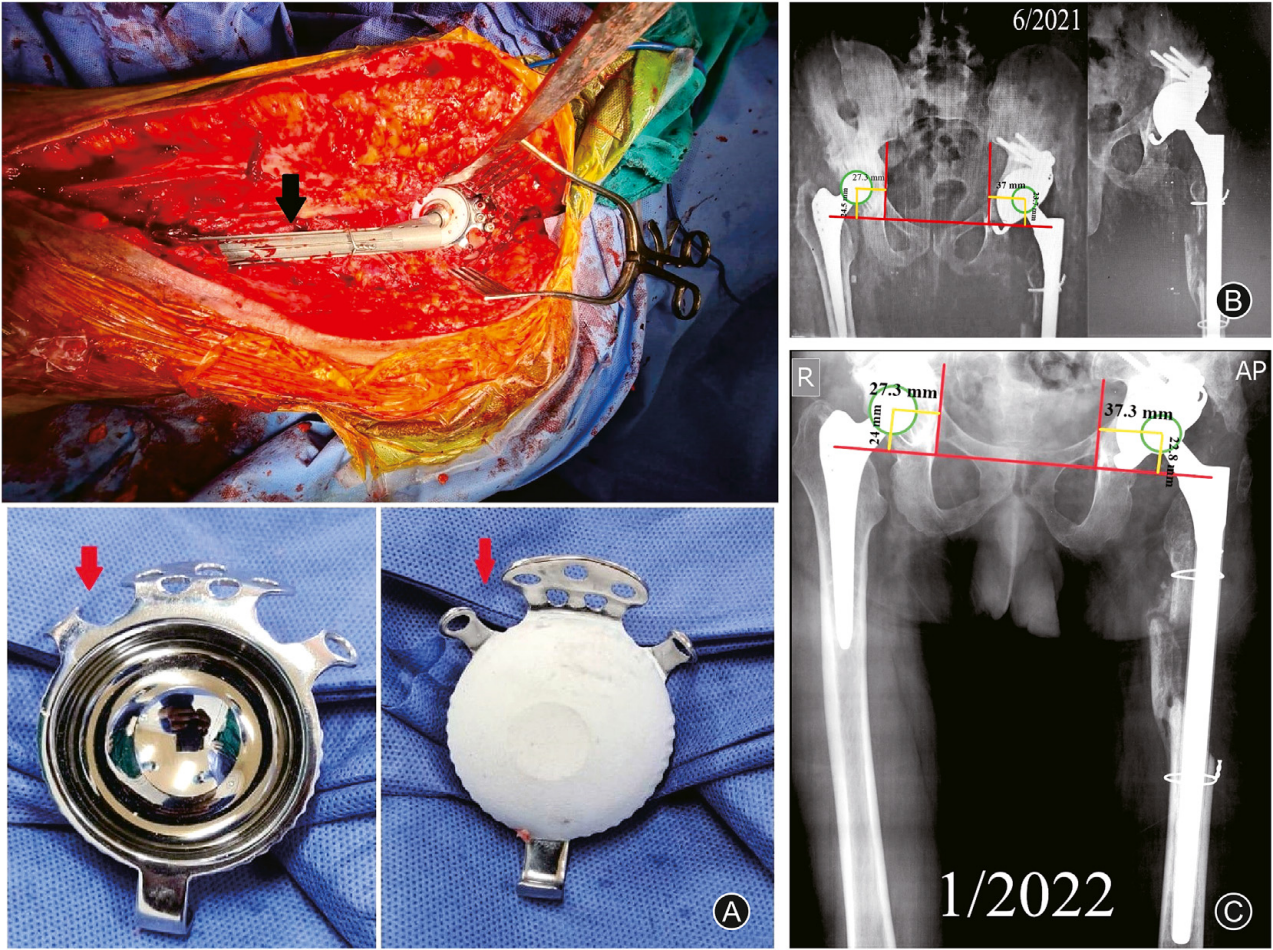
Last revision of the left total hip arthroplasty. (A) Intraoperative images showing the Wagner cementless stem (black arrowhead) and cementless dual mobility cup (red arrowhead); (B) Immediate postoperative radiographs; (C) Eight months follow-up radiographs.
